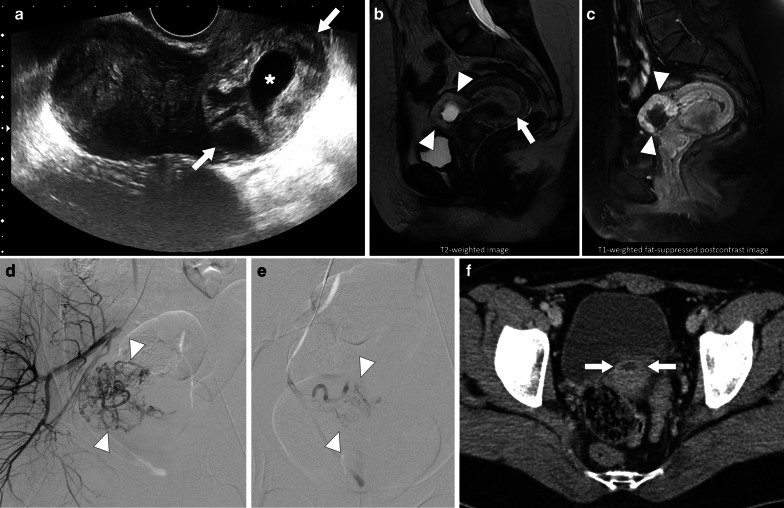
Ectopic pregnancy in the cesarean scar. A 29-year-old female patient presenting with recent onset post-coital bleeding. Gynecological examination was suspicious for a cervical mass. Serum beta-hCG level was mildly elevated (2100 mIU/mL). a Transvaginal gray scale US examination clearly outlined a mass (arrows) with central cystic component (asterisk) and thick walls. There were no detectable fetal elements nor fetal cardiac activity within the cystic component of the lesion. b, c Sagittal plane T2W and T1W postcontrast images, respectively, better demonstrate the same lesion with central cystic component and intense enhancement in its thick walls (arrowheads). Also note was made of thick endometrial lining suggestive of decidual reaction (arrow). The imaging and laboratory findings were inconclusive but a conservative approach was elected as the patient expressed her desire to preserve fertility. Oral methotrexate was started and a simultaneous endoarterial embolization was performed. d Intense vascularity was noted at the site of the lesion in pre-embolization angiography (arrowheads). e The lesion becomes completely devascularized after embolization (arrowheads). Her recovery was uneventful and serum beta-hCG levels precipitously dropped after these treatments. f Axial plane postcontrast abdominal CT image 2 year after the initial presentation showed a small residual cystic lesion (arrows), and confirmed almost complete regression of this mass
